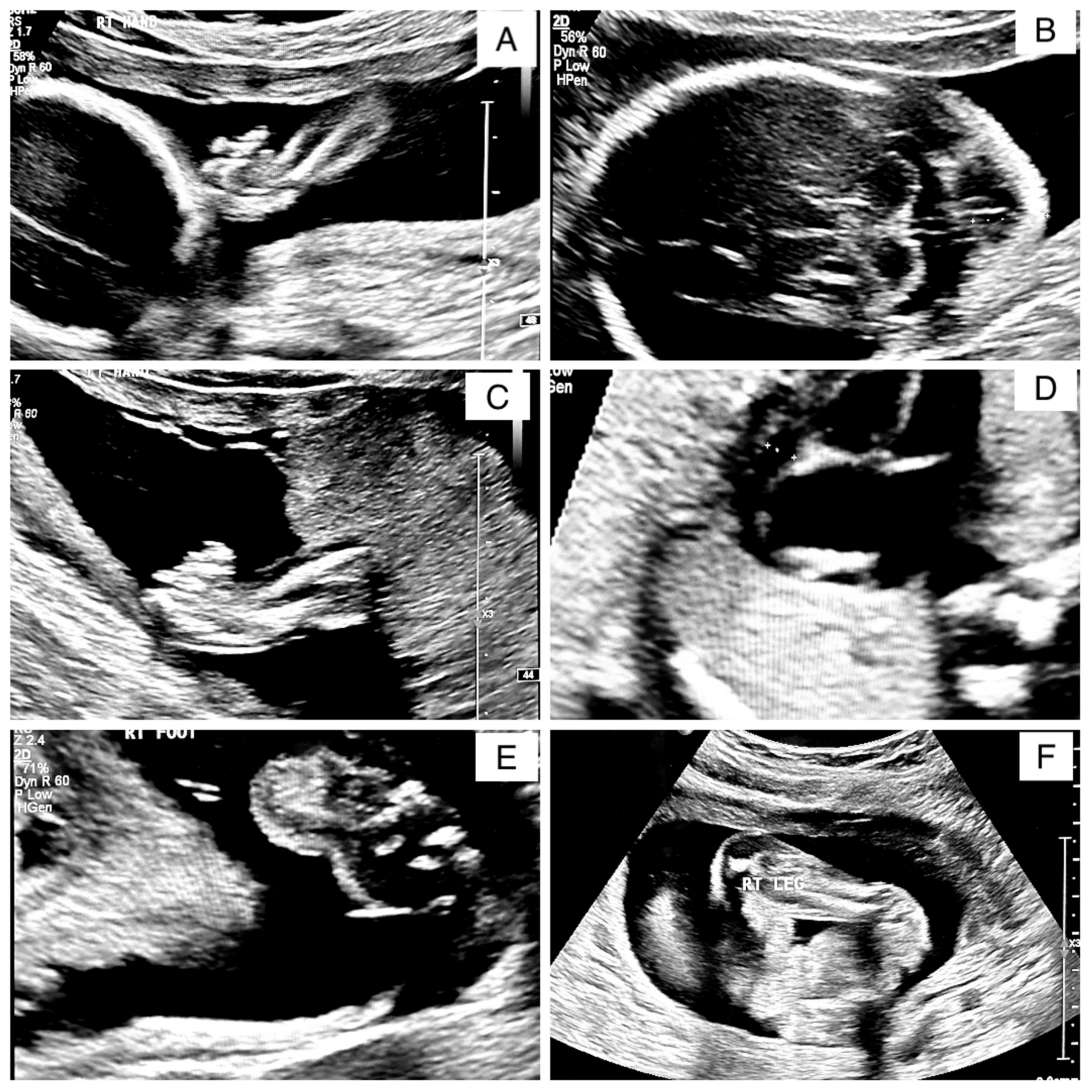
Ultrasound scan at 20 weeks gestation (A) The right upper extremity appears to be shortened and the foot angle appears to be abnormal; (B) Thickened nuchal fold that was measured to be 11.94 mm; (C) The left hand is shown, appears to be in a clenched position; (D) Four chamber of the heart view. A pericardial effusion with an abnormal heart axis; (E) The right foot angle presents in an abnormal fashion suggesting club foot; (F) The right lower extremity appears to be shortened.
